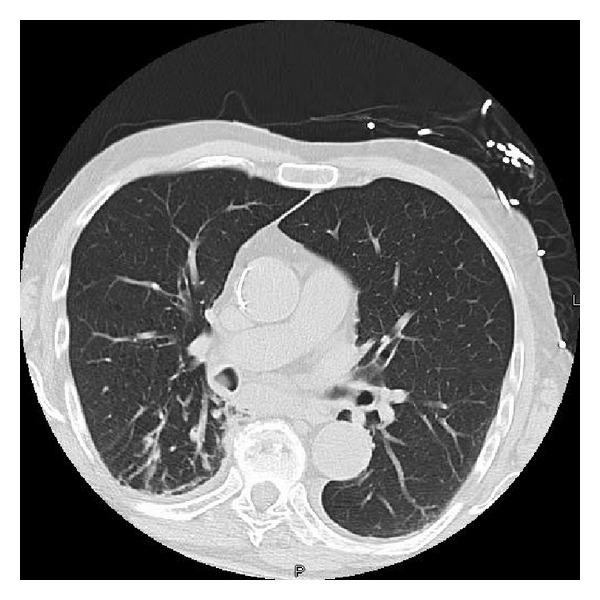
CT scan of chest showing a debris/possible endobronchial lesion in the bronchus intermedius of right lung.
